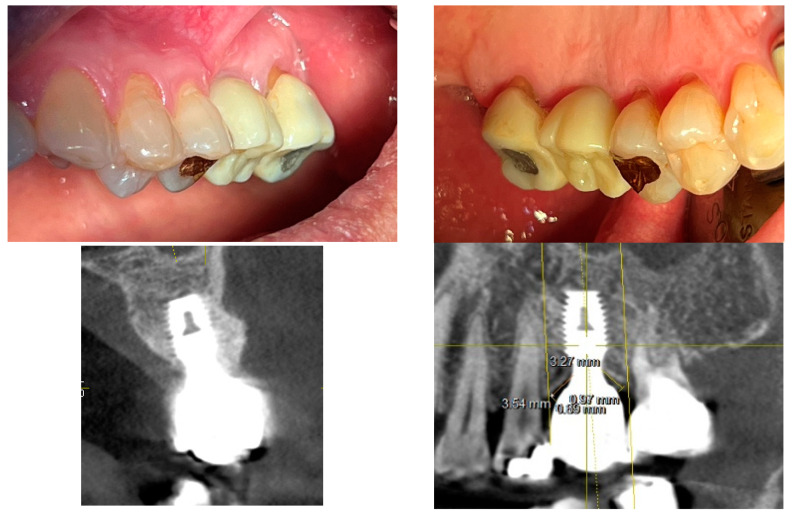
Clinical and radiographic outcomes of an SPI-restored upper left first molar. Upper Two Images (Clinical Photos): Show the restored upper left first molar with well-integrated soft tissue, demonstrating a natural emergence profile and coronal flaring of the implant restoration. These features contribute to both biological stability and esthetic success. Lower Left Image (CBCT Cross-Section, Buccolingual View): Displays the implant placement. While the PCO effect suggests that the buccal margin is positioned more apically than the mesiodistal margin—resulting in a shorter overall distance from the fixture–abutment connection (FAC) to the soft tissue margin in the buccal aspect—this does not necessarily imply that the crestal zenith at the buccal side must always be positioned apically. Unlike the palatal side, where the crest may be observed at an epicrestal level, implant placement at the buccal side can still be subcrestal, provided it meets the required vertical depth for peri-implant stability or is intended to enhance the bone phenotype. Lower Right Image (CBCT with Measurements): Highlights the transitional zone length (TZL) and soft tissue thickness (STT), showing the vertical and horizontal dimensions of the peri-implant soft tissue. Additionally, it reflects the influence of the mesiodistal crestal slope (MDCS), emphasizing how ridge morphology affects implant positioning, peri-implant soft tissue stability, and overall emergence profile.
